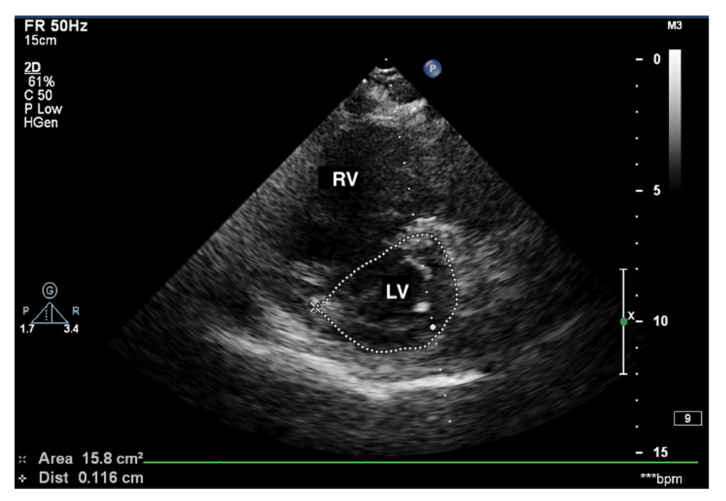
D-sign, enlarged right ventricle, ventricular septum displaced towards LV. 2D-TTE, short-axis view (SAX). LV: left ventricle; 2D-TTE: two-dimensional transthoracic echocardiography.
